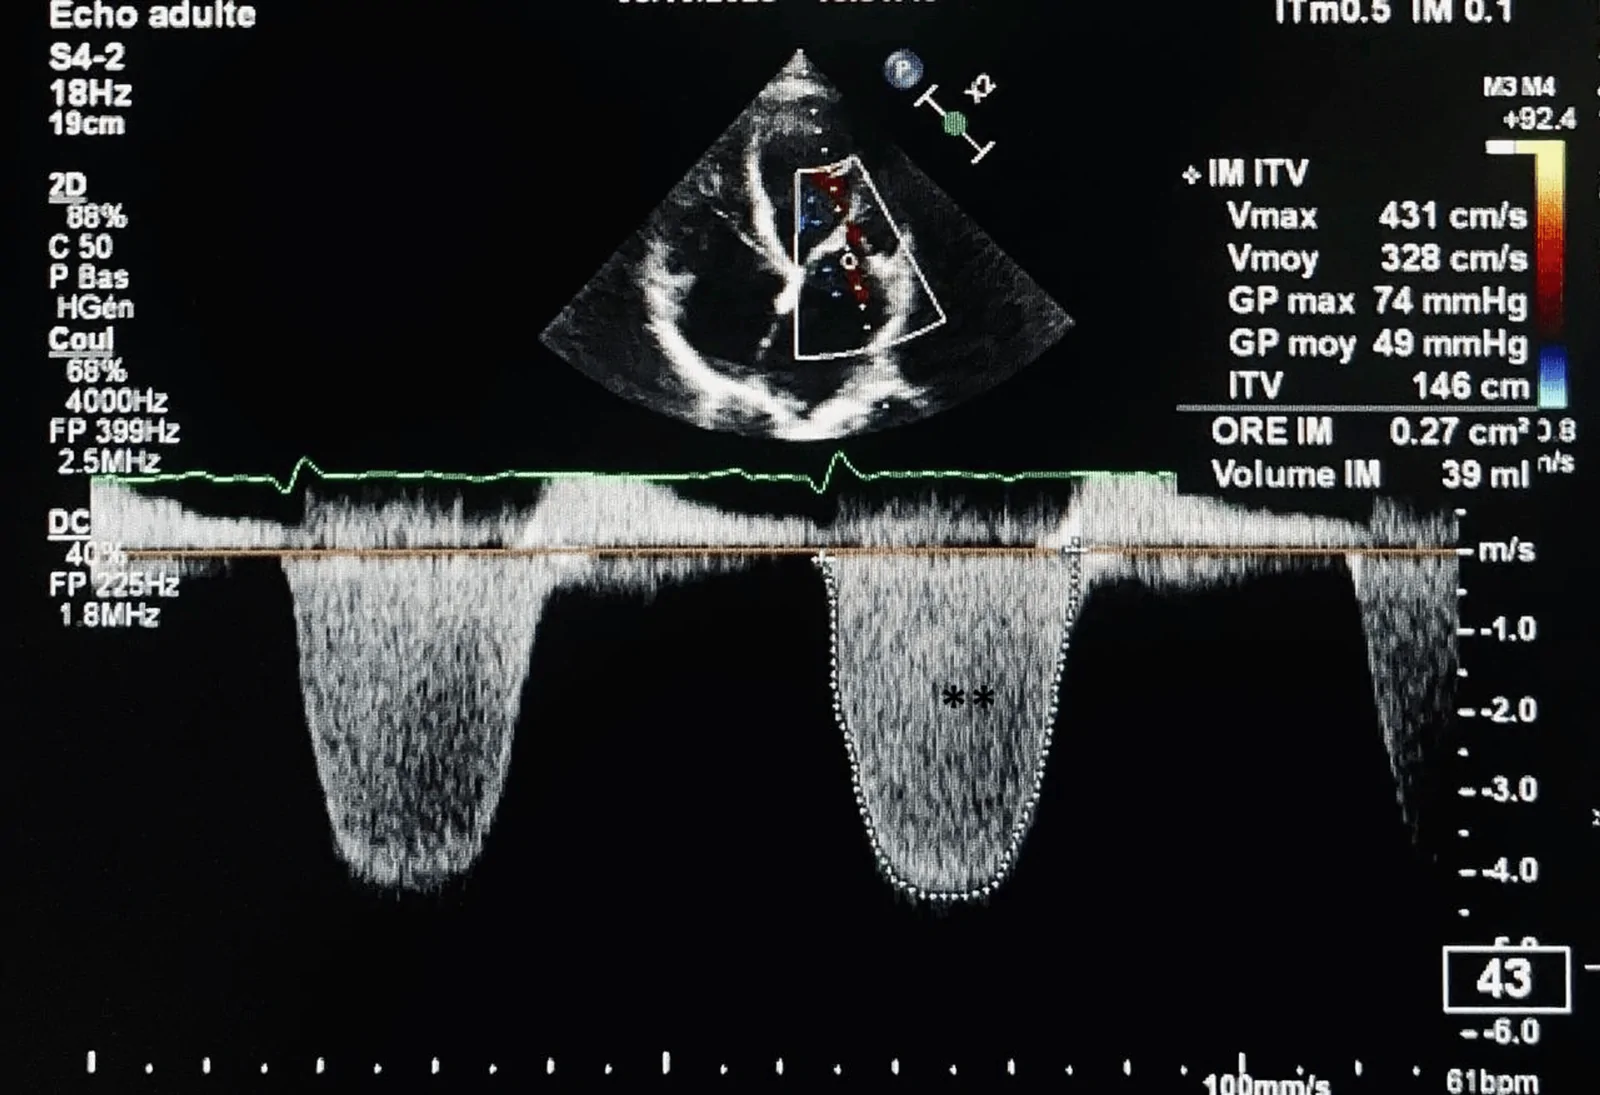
Apical four-chamber view of Doppler assessment of mitral regurgitation, showing on CW Doppler a dense and parabolic jet (double asterisk). VTI: Volume time integral; CW: continuous wave; mitral VTI/aortic outflow VTI ratio = 1.21.
